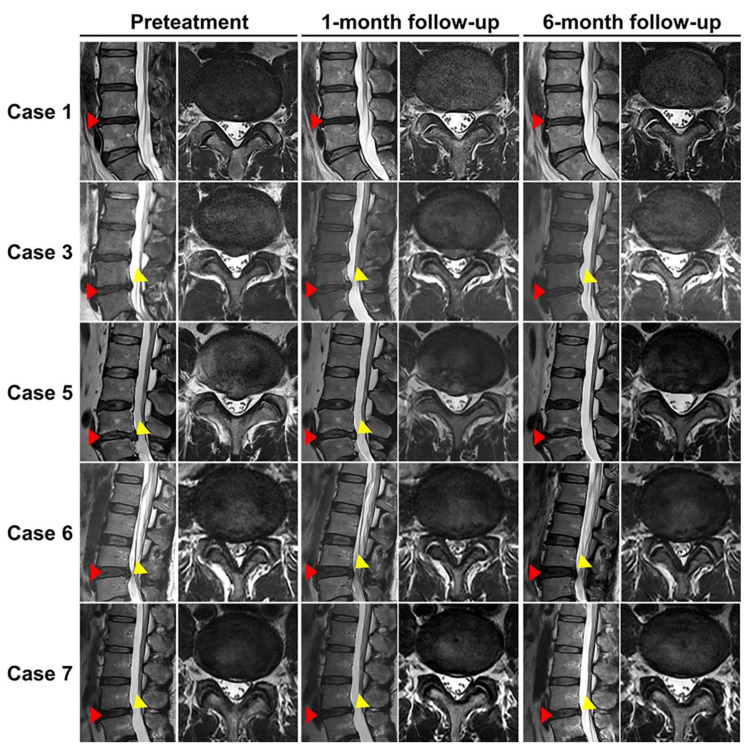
Assessment of disc degeneration grade based on the evaluation of sagittal and axial T2-weighted images. Red triangle indicates the treated L4-5 disc. Yellow triangle indicates disc herniation.
